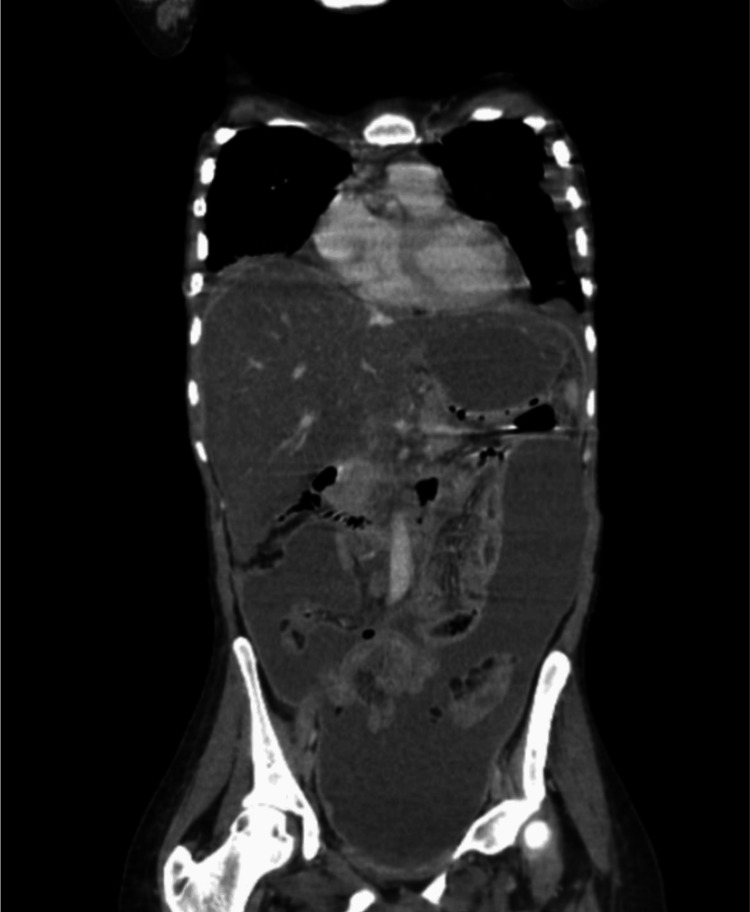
Coronal CT - ascites, pneumoperitoneum, and centrally located bowel loops
